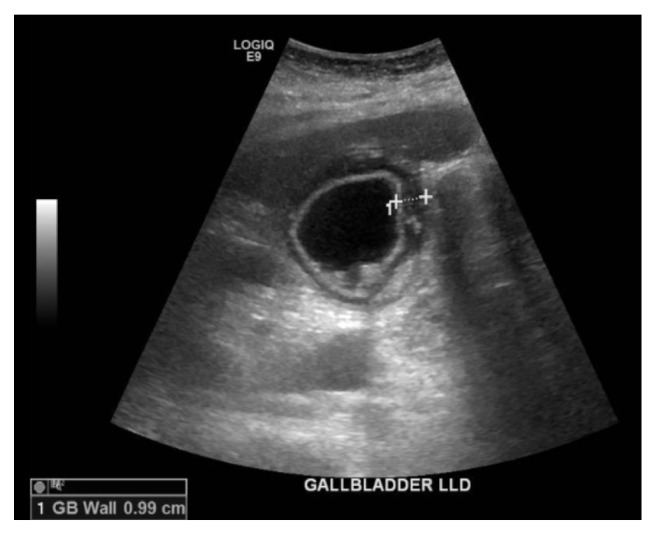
Ultrasound of gallbladder showing inflamed gallbladder with multiple nonmobile stones with shadowing in the gallbladder body. The gallbladder wall is thickened to 0.99 cm. A moderate amount of pericholecystic fluid is identified.
